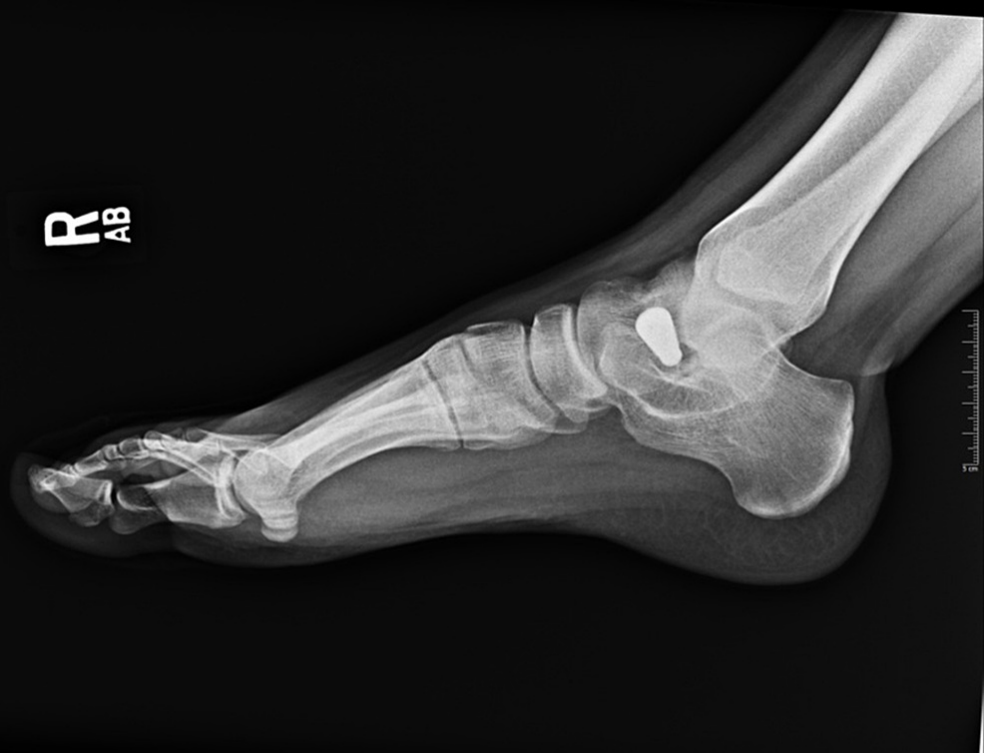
Lateral postoperative right foot weight-bearing X-ray.
